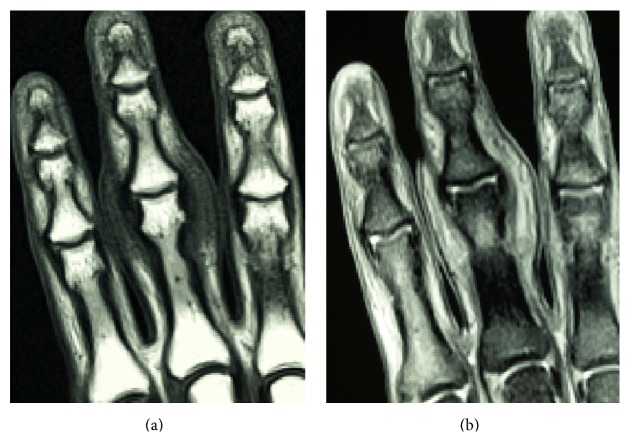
The lesion indicated homogenous isointensity to the muscle on a T1-weighted image (a). On the postcontrast fat-saturated T1-weighted image, the lesion was heterogeneously hyperintense, and no other abnormal enhancement was noted (b).
